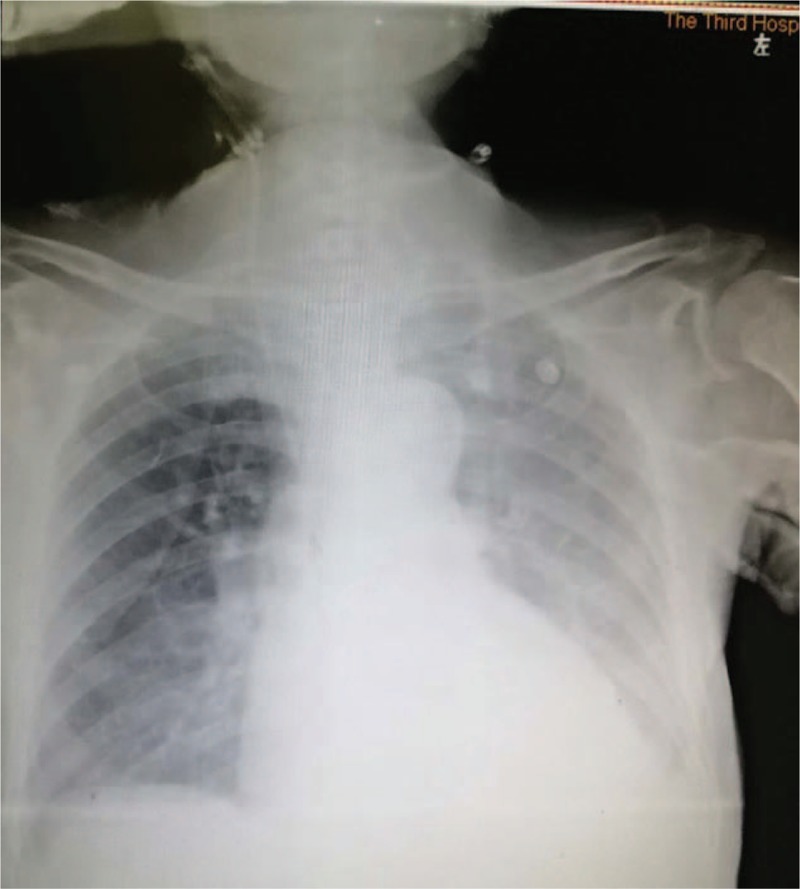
Bedside chest X-ray showed an enlarged heart.
